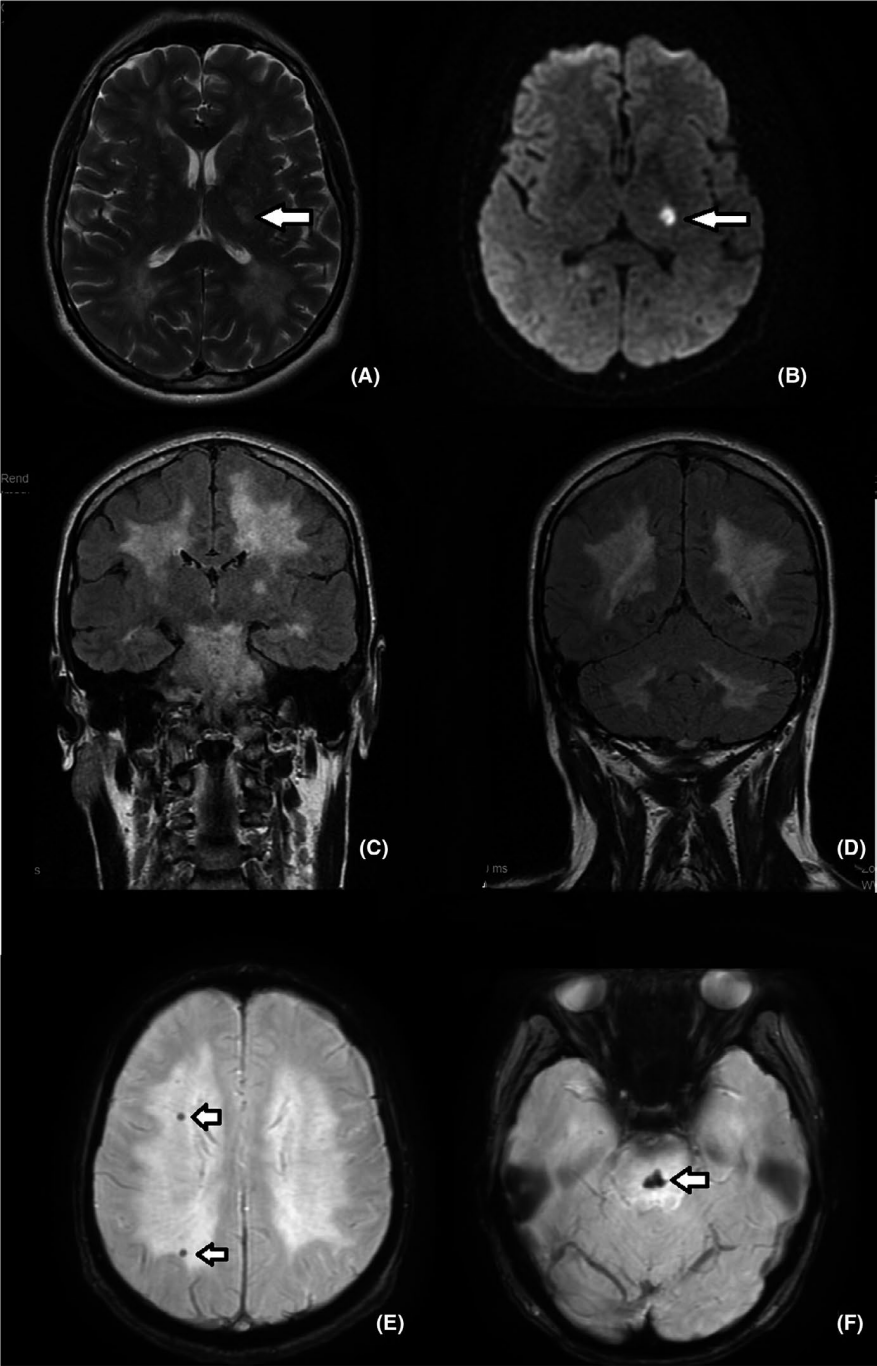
A, Axial cross‐section MRI image of the brain, T2 weighted, revealing diffuse white matter hyperintensities. B, DWI sequence. Arrows indicate to a hyperintense lesion located in the posterior limb of the left internal capsule (A, B). C, D, Coronal cross‐section MRI images on FLAIR sequence, revealing supratentorial and infratentorial white matter hyperintensities. E, F, SWI sequence. Arrows indicate to hemosiderin deposits in the centrum semiovale (E) and the pons (F)
